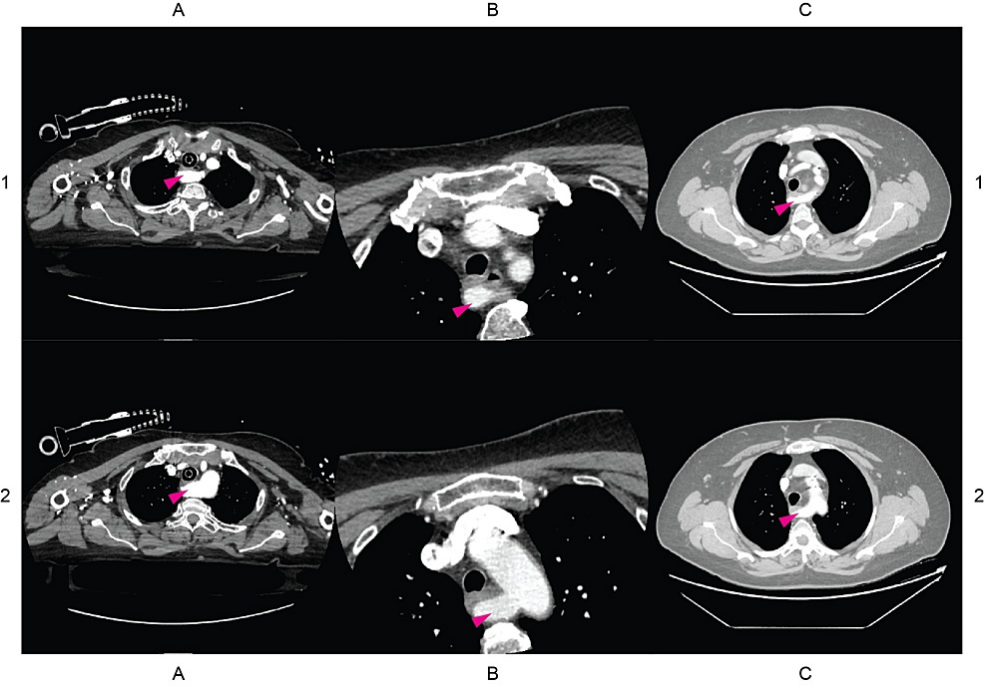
Axial CT images of patients with identifiers ID036, ID230, and ID587 Columns A, B, and C contain raw axial CT images of patients with identifiers ID036, ID230, and ID587 respectively. The crosshairs on the left are for orienting the corresponding row. The magenta arrowheads label the aberrant right subclavian arteries, also called lusorian arteries. Each patient has a retroesophageally traversing lusorian artery resulting in an anterior-posterior compression of the esophagus. Nota Bene (NB): in the C1 image the lower pole of the left thyroid lobe spreads into the thorax approaching the trachea, the esophagus, and the lusorian artery.
